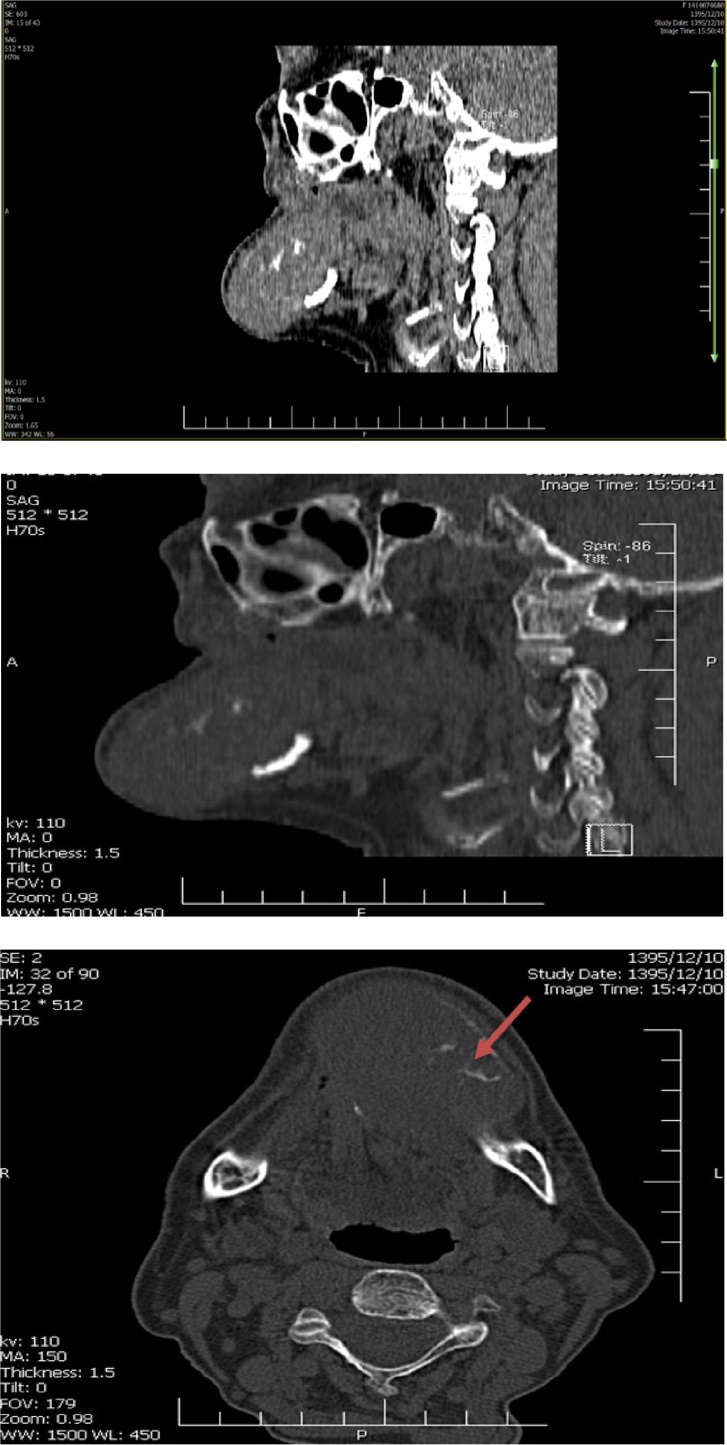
Spiral mandible CT scan without contrast: A well-defined, lytic expansle lesion in mandibular body (arrow).
